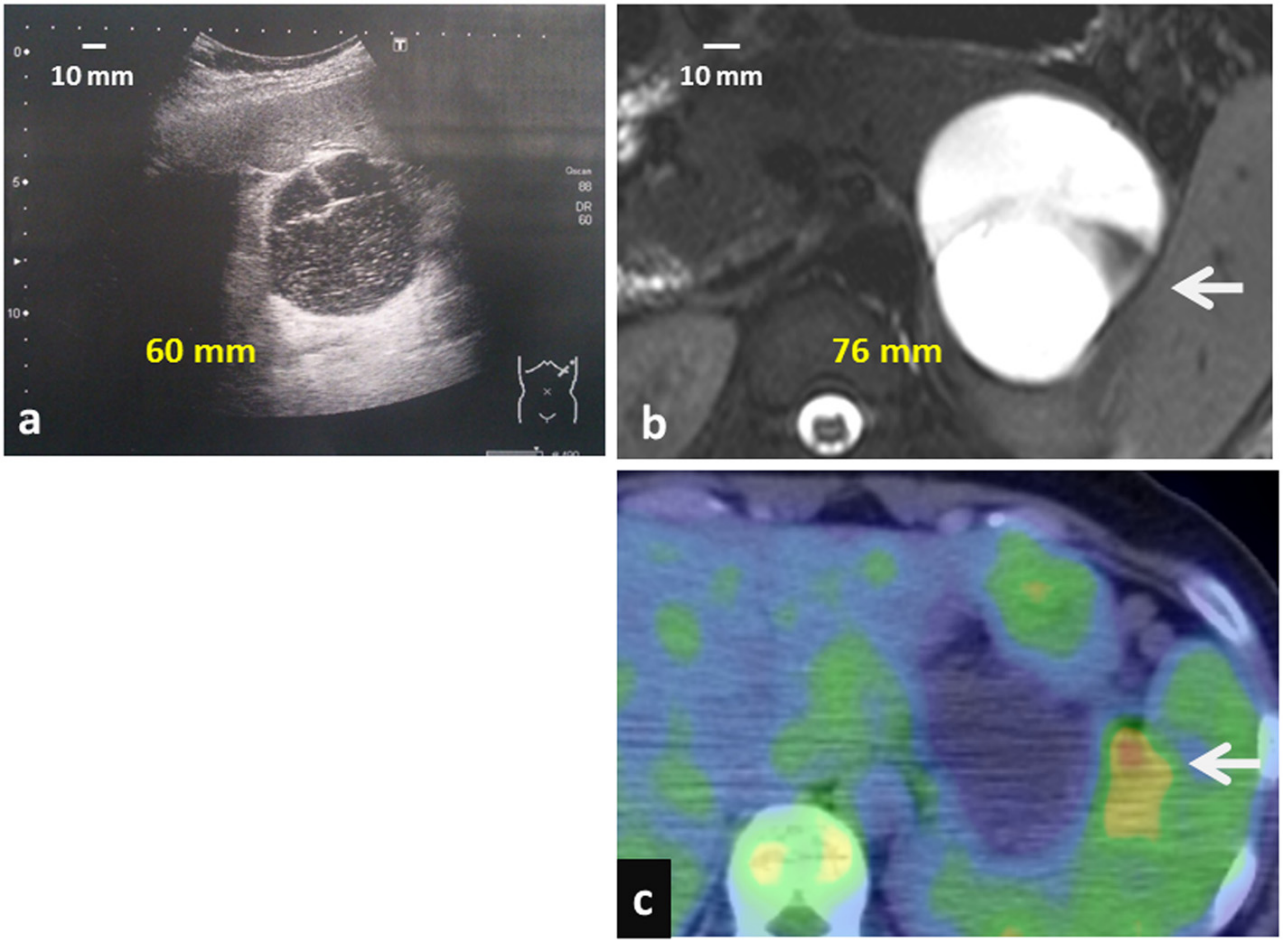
Imaging studies. (a) A 60-mm cystic mass was detected in the body and tail of the pancreas without a mural nodule or thickening of the wall by abdominal ultrasound (during the fourth month of gestation). (b) Magnetic resonance imaging (MRI) showed a 76 × 69-mm cystic tumor (arrow), in which the contents were hyperintense in T2-weighted imaging. There were septa in the tumor, and one part of it was thickened and enhanced. (c) Fluorodeoxyglucose positron emission tomography-computed tomography (FDG-PET/CT) showed high uptake (arrow) in part of the cystic tumor (SUVmax 3.03).
